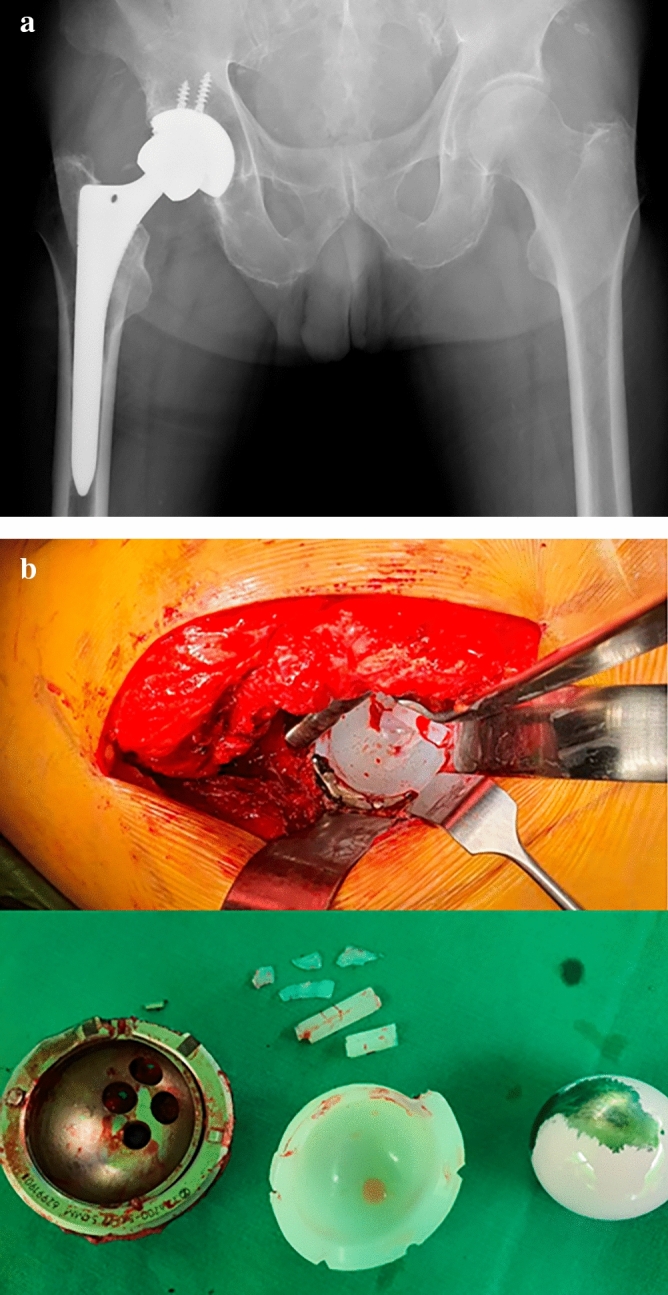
(a) Plain radiograph showing eccentric elevation of prosthetic femoral head. (b) Intraoperative photographs of a liner rim fracture.
